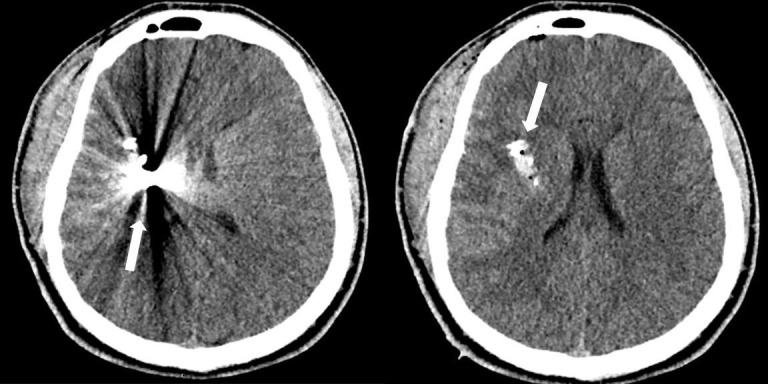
Presenting computed tomography (CT) head without contrast reveals penetrating head injury from high-velocity projectile with retained projectile adjacent to the right basal ganglia.
